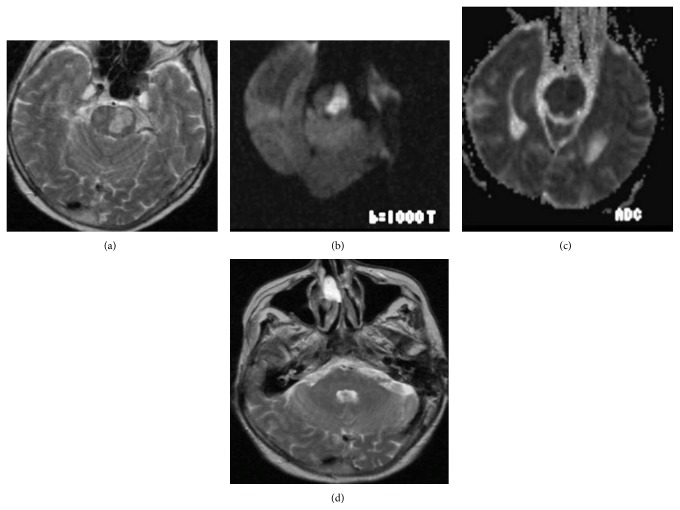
Left paramedian acute pontine infarction: axial T2WI (a) hyperintense signal on the left side of the pons, axial DWI (b) and ADC maps (c) restricted diffusion, and axial T2WI (d) MCPs were normal.
